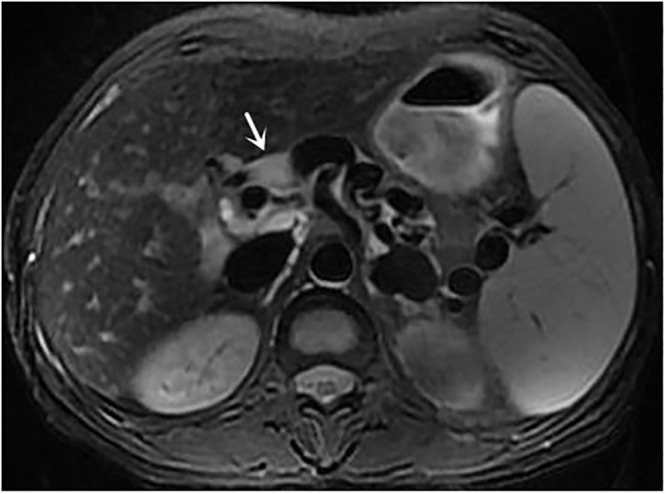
female, 52 years, axial T2-weighted MRI shows lymphadenopathy (*arrow*), and portosystemic collaterals are shown as areas without signal intensity.
